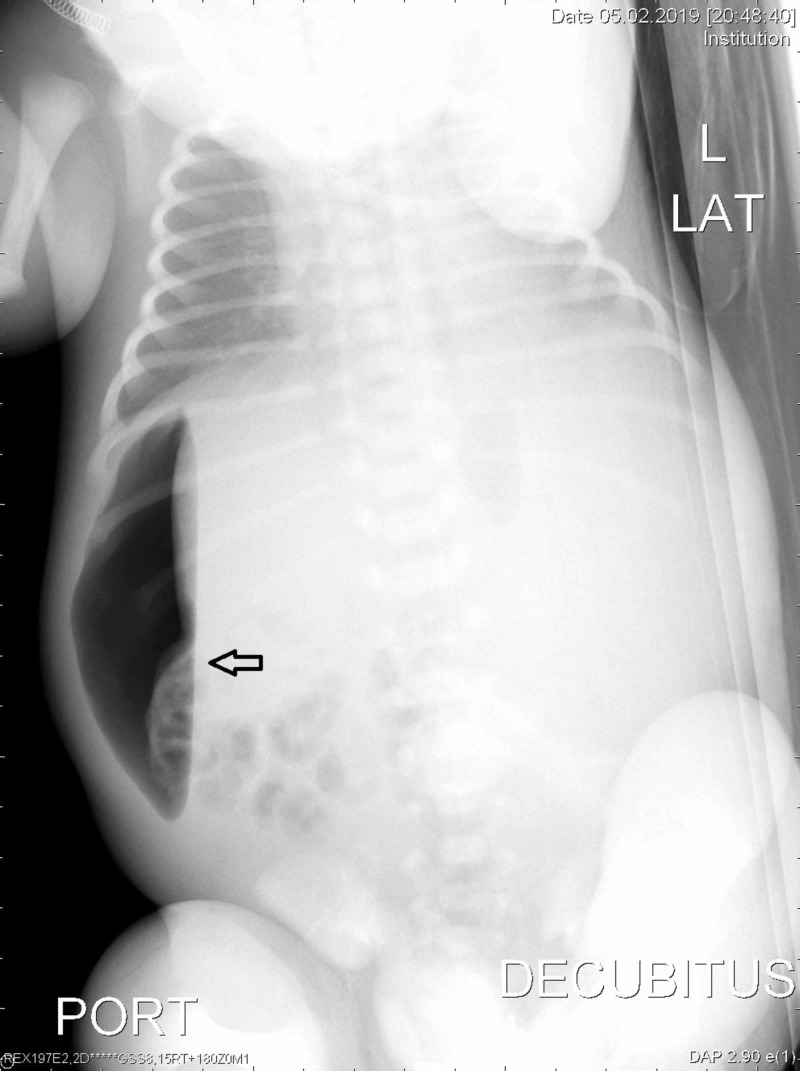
X-ray of abdomen lateral decubitus. Arrow showing free gas along the right half of abdomen
